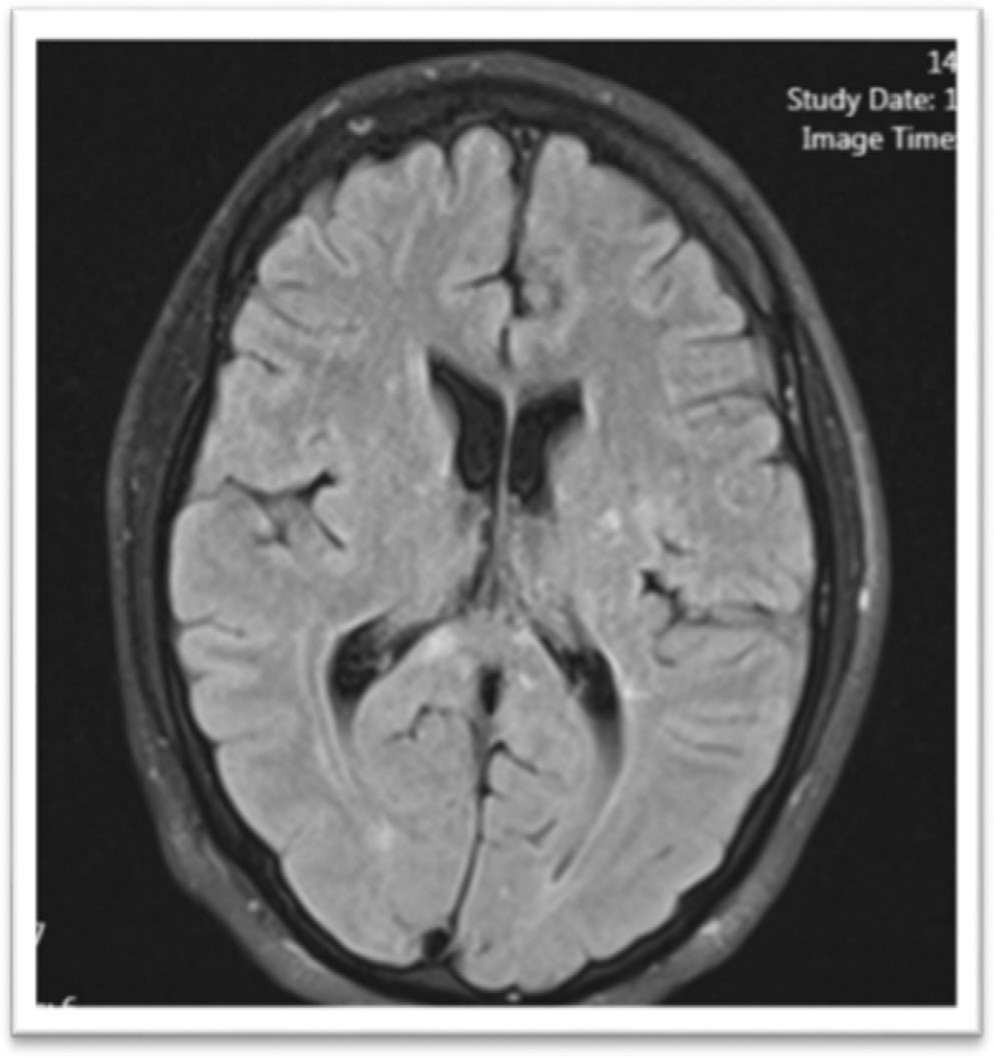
MRI on current admission. Axial FLAIR brain MRI reveals typical, stable MS plaques with no new enhancing lesions to explain the patient's acute neurological deterioration.
